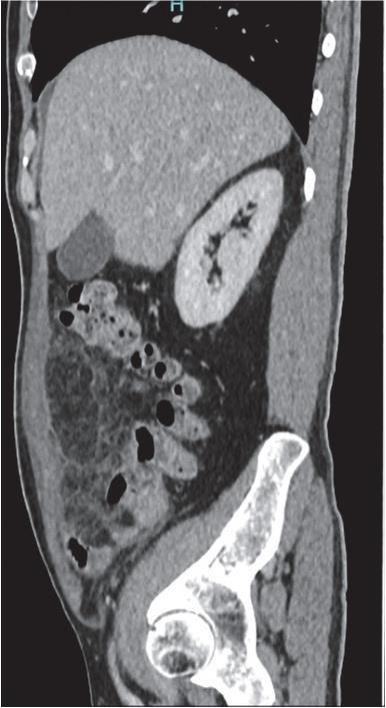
CT sagittal view. CT abdomen and pelvis. Increased fat stranding of omentum along anterior aspect of ascending colon and inferior to hepatic flexure measuring 6 cm × 10 cm × 10 cm (A P × W × CC) with adjacent peritoneal thickening and enhancement with evidence of thrombosed mesenteric vessels.
